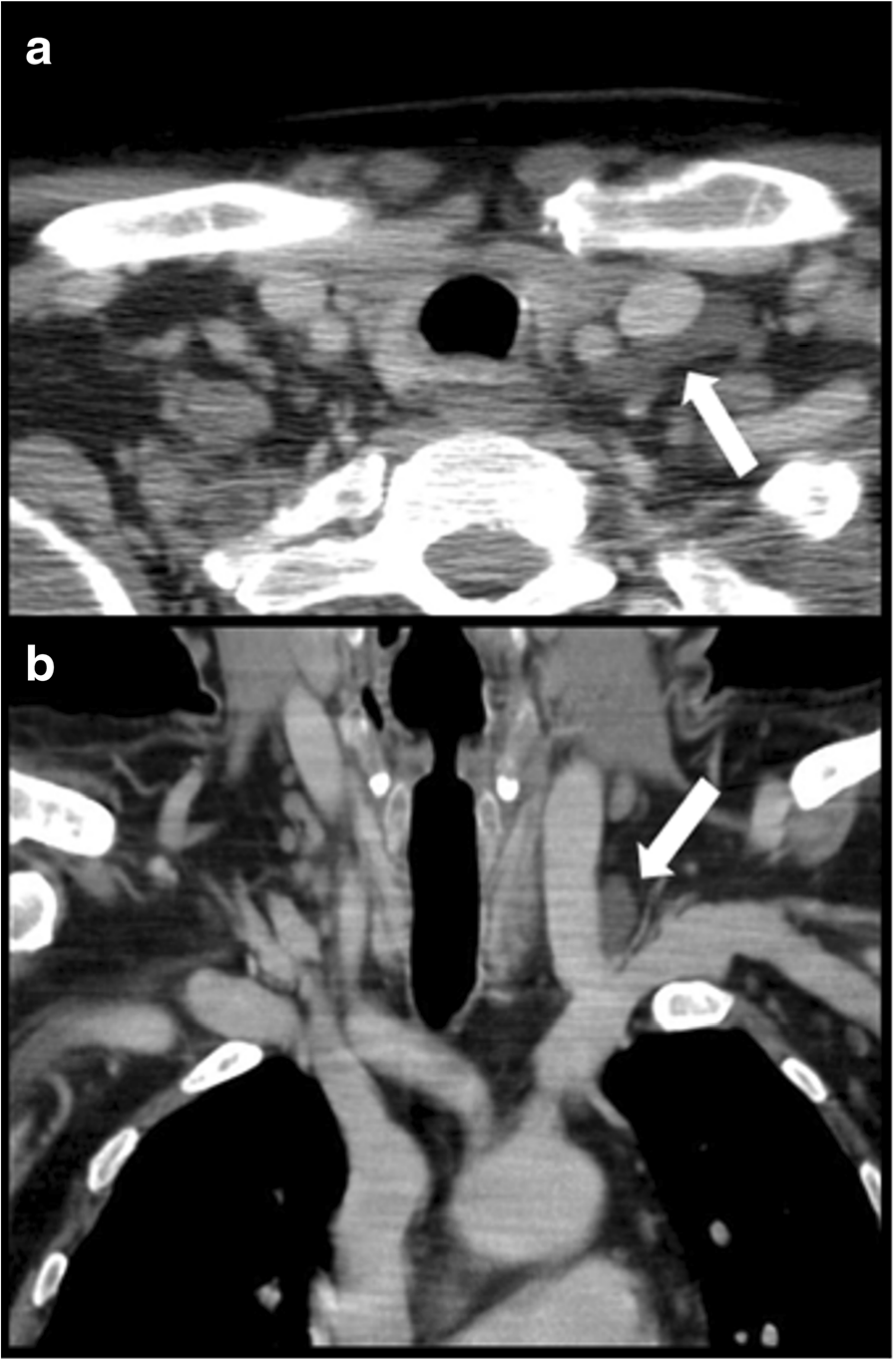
A 66 year old male with pharyngeal lymphoma. Contrast enhanced computed tomography in the axial (a) and coronal (b) plane. Tubular (axial plane) configuration of the thoracic duct (arrows)
